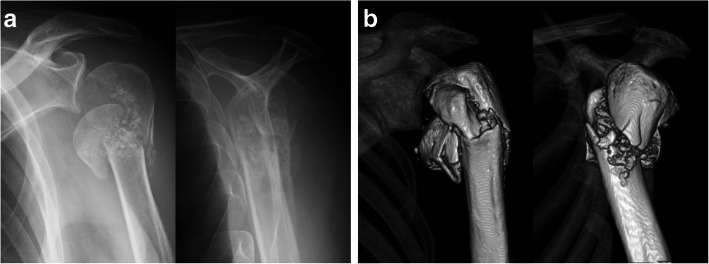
Left proximal humeral fracture of a 53-year-old man. The humeral head is displaced inferiorly with the other components staying together. The consensus of assessments is divided from 2-part fracture to 4-part fracture with the conventional Neer classification, but it is seen as a 4-part fracture with the modified classification. a Antero-posterior and scapular-Y views of plain radiographs. b Anterior and lateral views of 3-dimensional CT reconstructions
